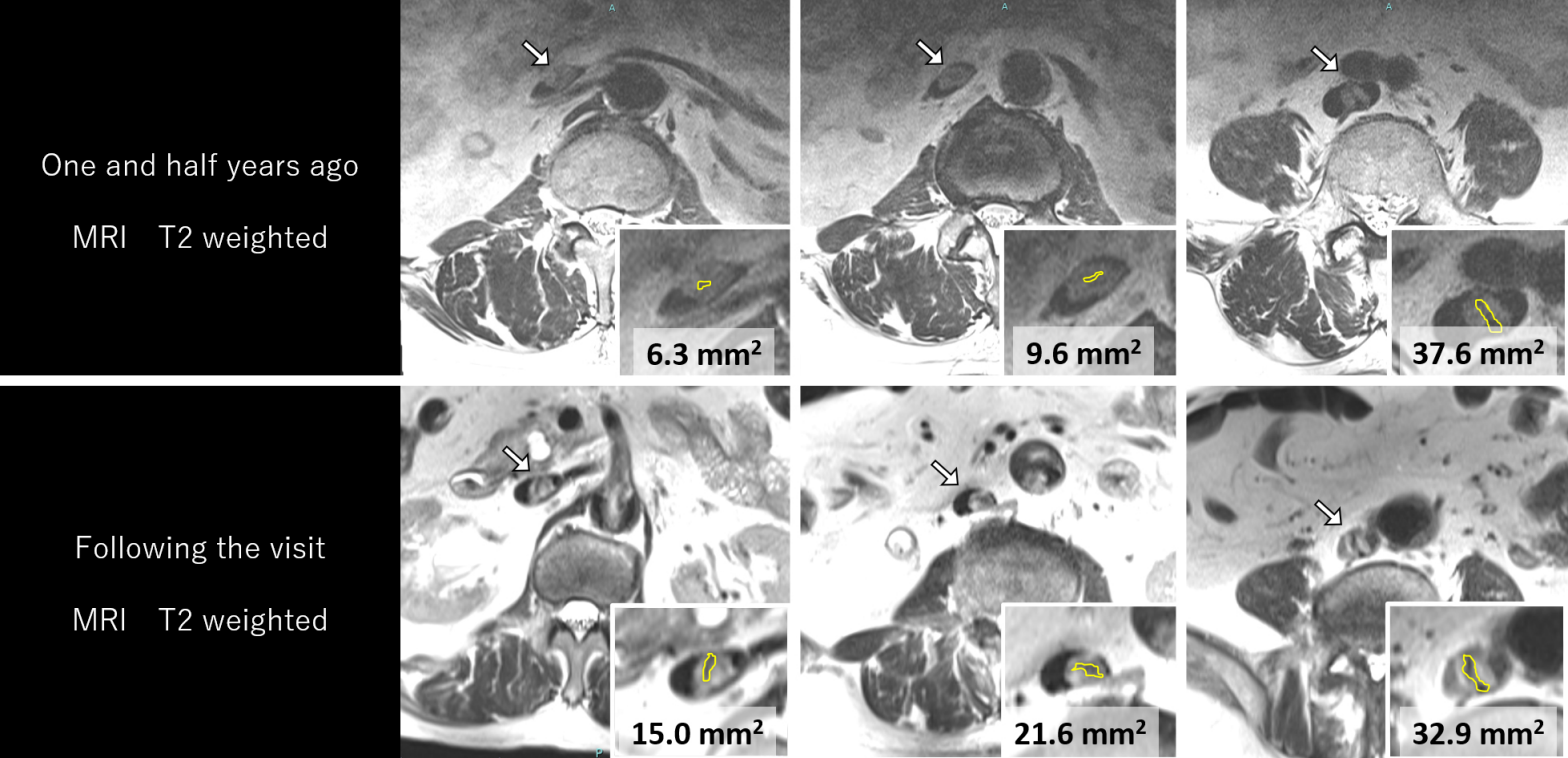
Increasing the low-intensity area (yellow enclosure and area value) over time by comparing magnetic resonance imaging performed 18 months ago and the day following the visit.
